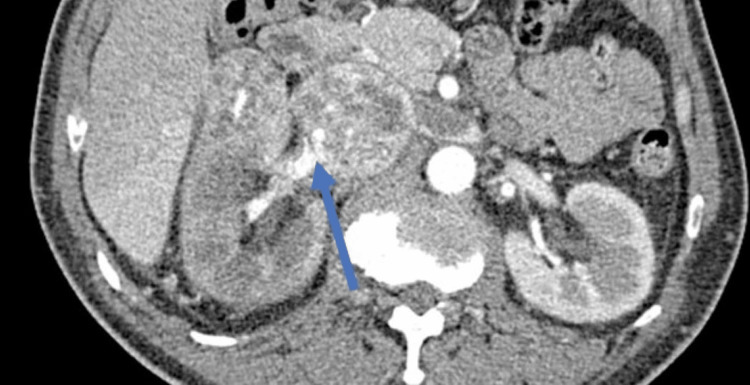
Contrast-enhanced CT showing right renal mass obliterating right renal vein and inferior vena cava.
